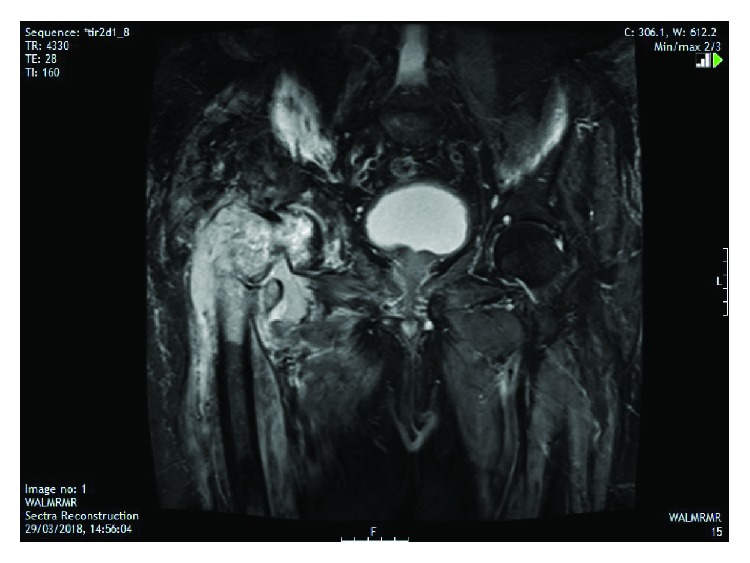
Magnetic resonance imaging demonstrating hyperintense marrow replacement, pathological fracture, and extraosseous soft tissue mass.
